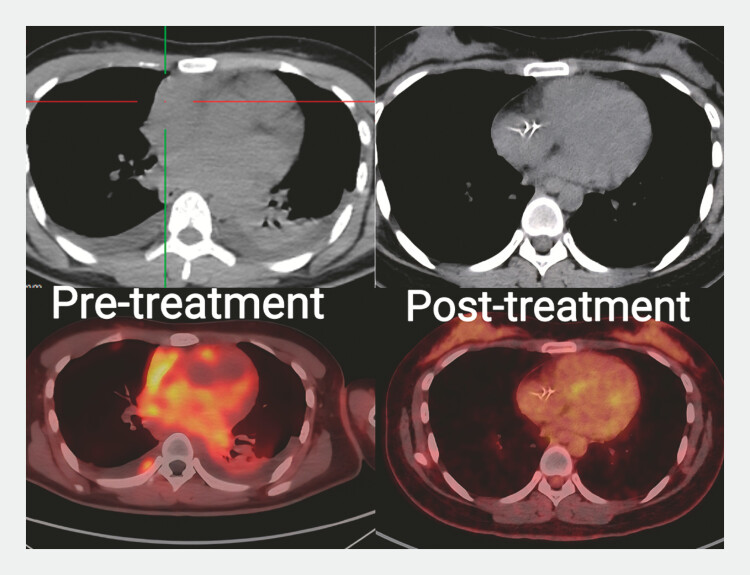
Five months after chemotherapy, the follow-up PET/CT showed significant improvement. PET/CT, positron emission tomography/computed tomography.
